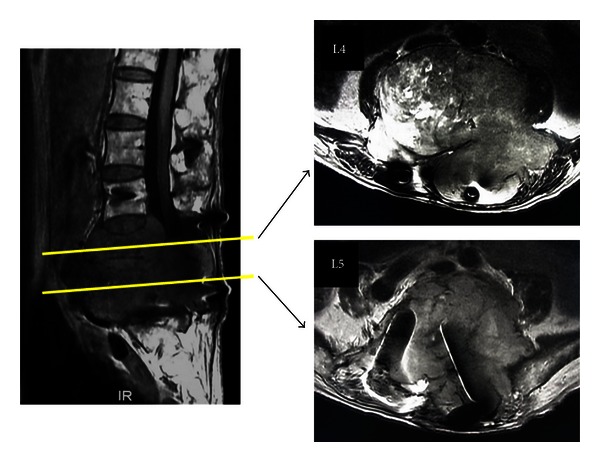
MRI before the second surgery (February 2010) revealed a large mass compressing the neural tissue at the L3, L4, and L5 levels.
